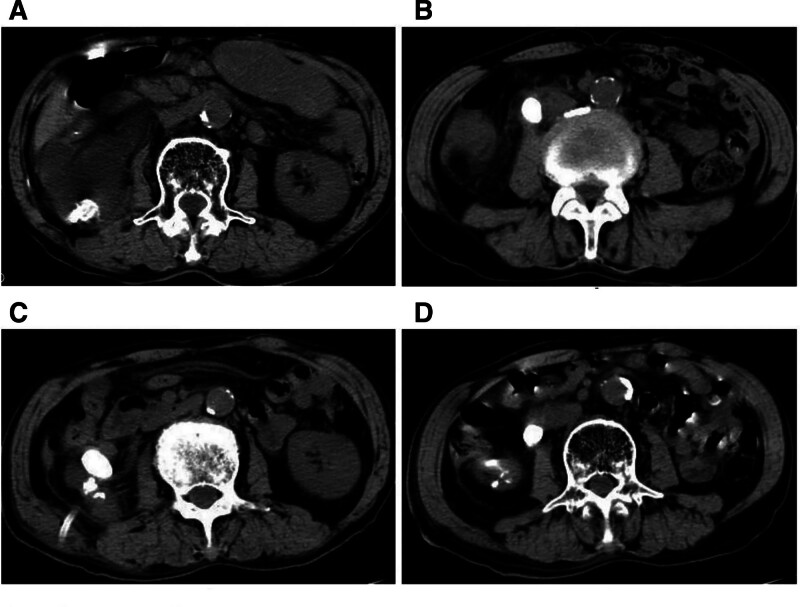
Preoperative imaging findings for nephrectomy. (A and B) CT imaging demonstrated right renal and proximal ureteral calculi with severe hydronephrosis; (C and D) Post-right nephrostomy CT scan showed atrophy of the right kidney with significant improvement in right hydronephrosis. CT = computed tomography.
